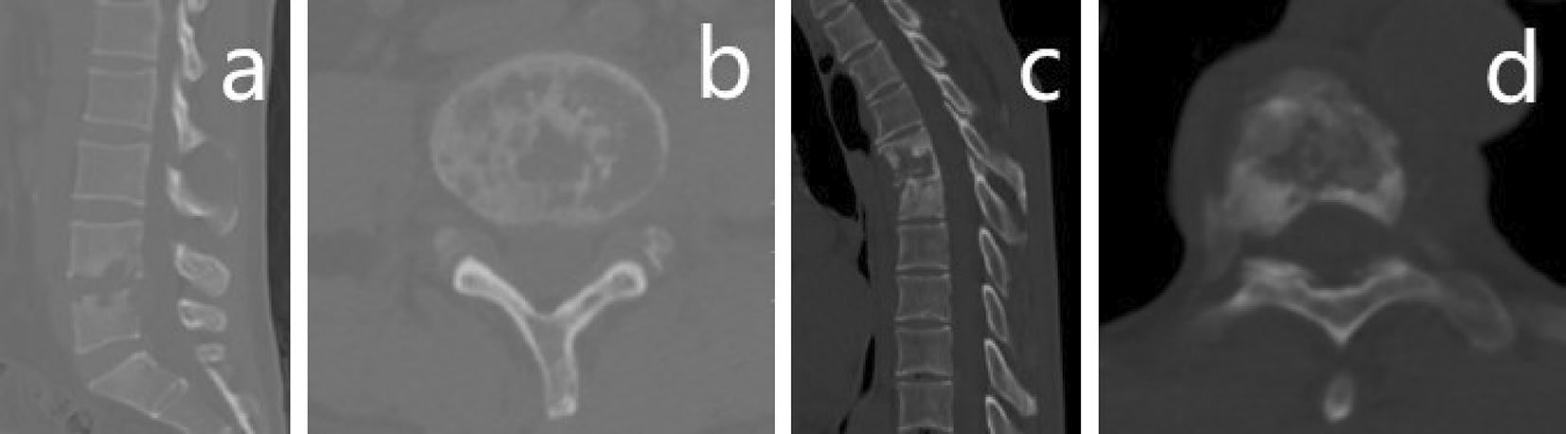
CT scan images of tuberculous spondylitis and pyogenic spondylitis. (a and b) CT scan images of pyogenic spondylitis. Lumbar spine involvement is more common in pyogenic spondylitis. (c and d) CT scan images of tuberculous spondylitis. Thoracic spine involvement is more common in tuberculous spondylitis.
